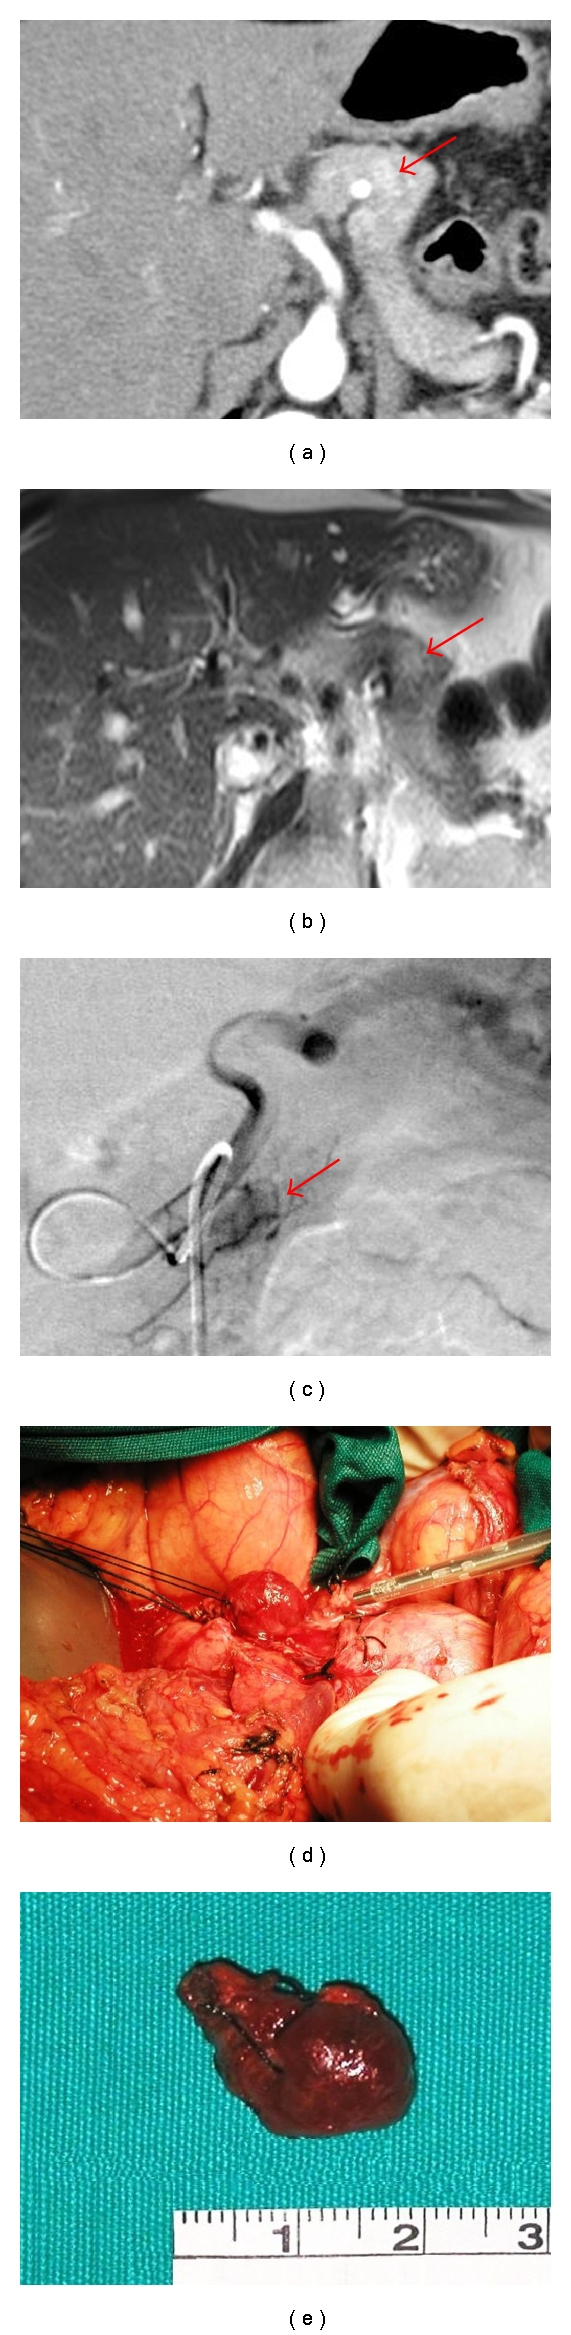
Imaging studies and intraoperative appearance. (a) A contrast-enhanced computed tomography slice of the upper abdomen, showing a mildly hyperintense nodule, about 1 cm in diameter, located between the body and the tail of the pancreas (red arrow). (b) A gadolinium-enhanced magnetic resonance slice of the upper abdomen, showing a mildly hyperintense nodule, about 1 cm in diameter between the body and the tail of the pancreas (red arrow), in the same location as identified by CT. (c) A digital subtraction angiography with the catheter positioned in the proximal splenic artery, showing nodular vascularization from the *arteria pancreatica magna* (red arrow). (d) Isolation and enucleation of the adenoma using the CUGA Excel system. (e) Macroscopic appearance of the adenoma soon after resection (scale bar in cm).
